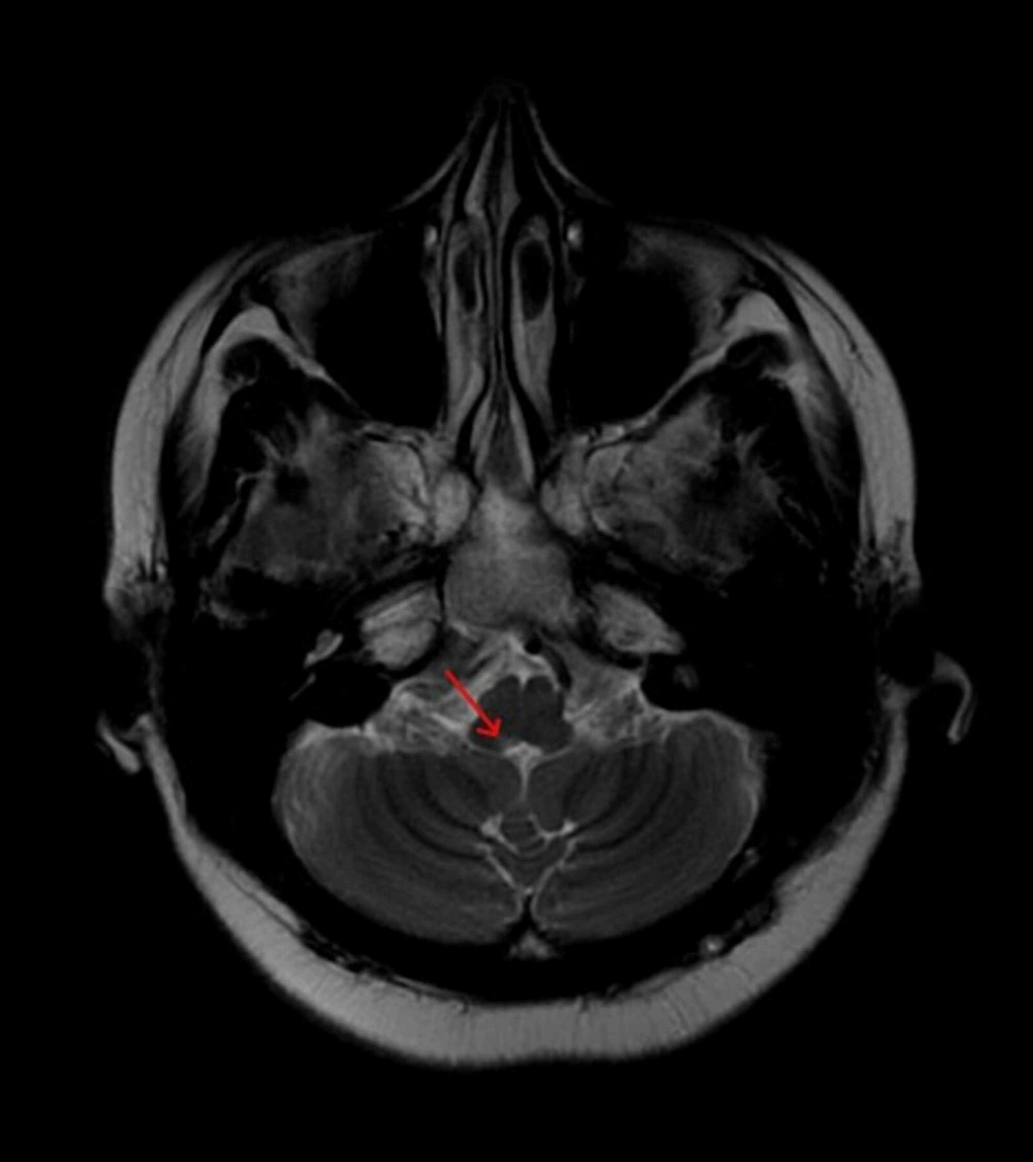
MRI brain showing a focus of active demyelination in the region of the dorsal medulla.
